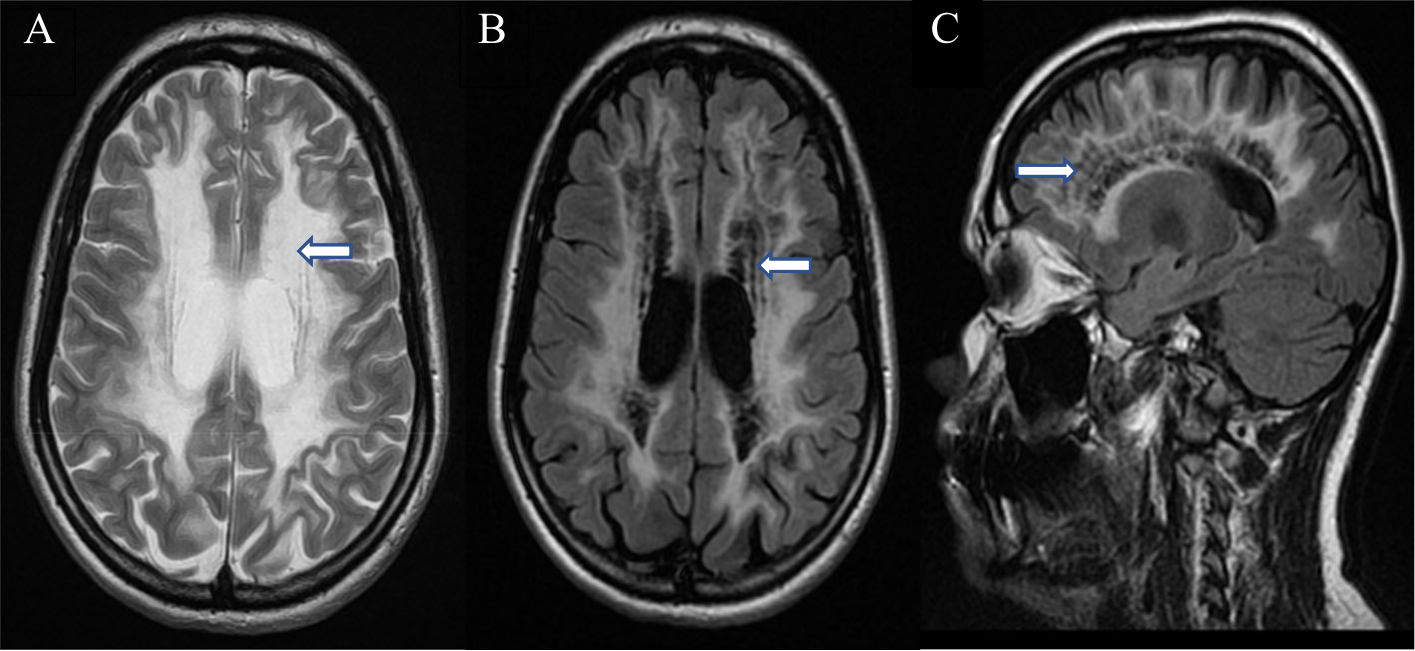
T1 and FLAIR images of the patient with vanishing white matter disease. a Axial T1-weighted image showing the diffuse abnormality of the white matter. b Axial FLAIR image showing the rarefaction and cystic degeneration of the white matter. c Sagittal FLAIR image showing the radiating stripe-like pattern within the white matter, indicative of residual tissue strands
